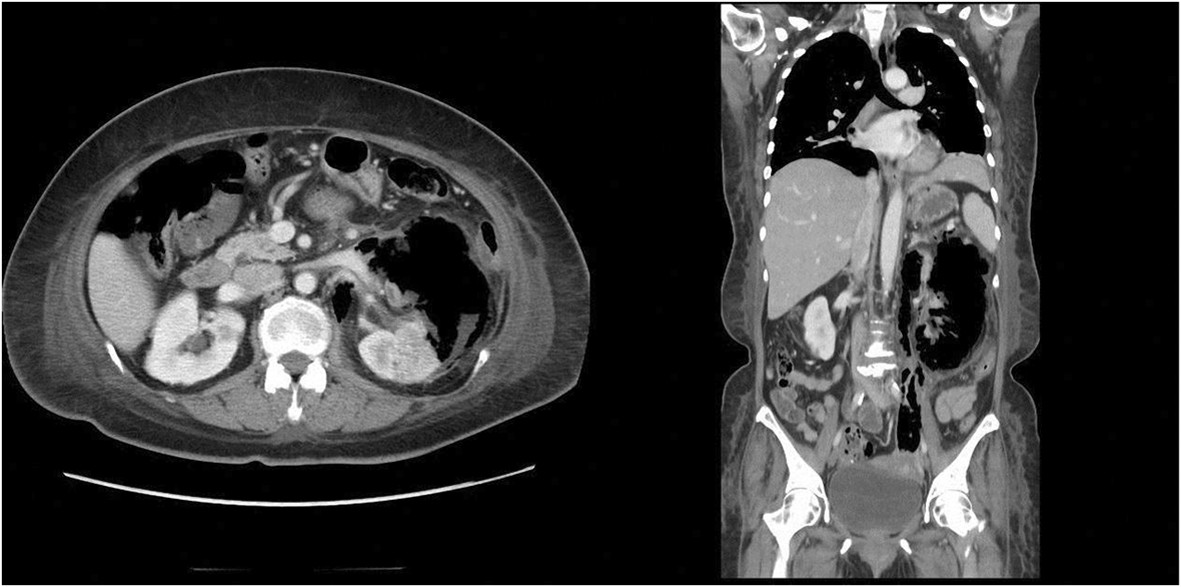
Contrast CT revealed the presence of gas in the left pelvicalyceal system with extending into paranephric area (Class 3B).
